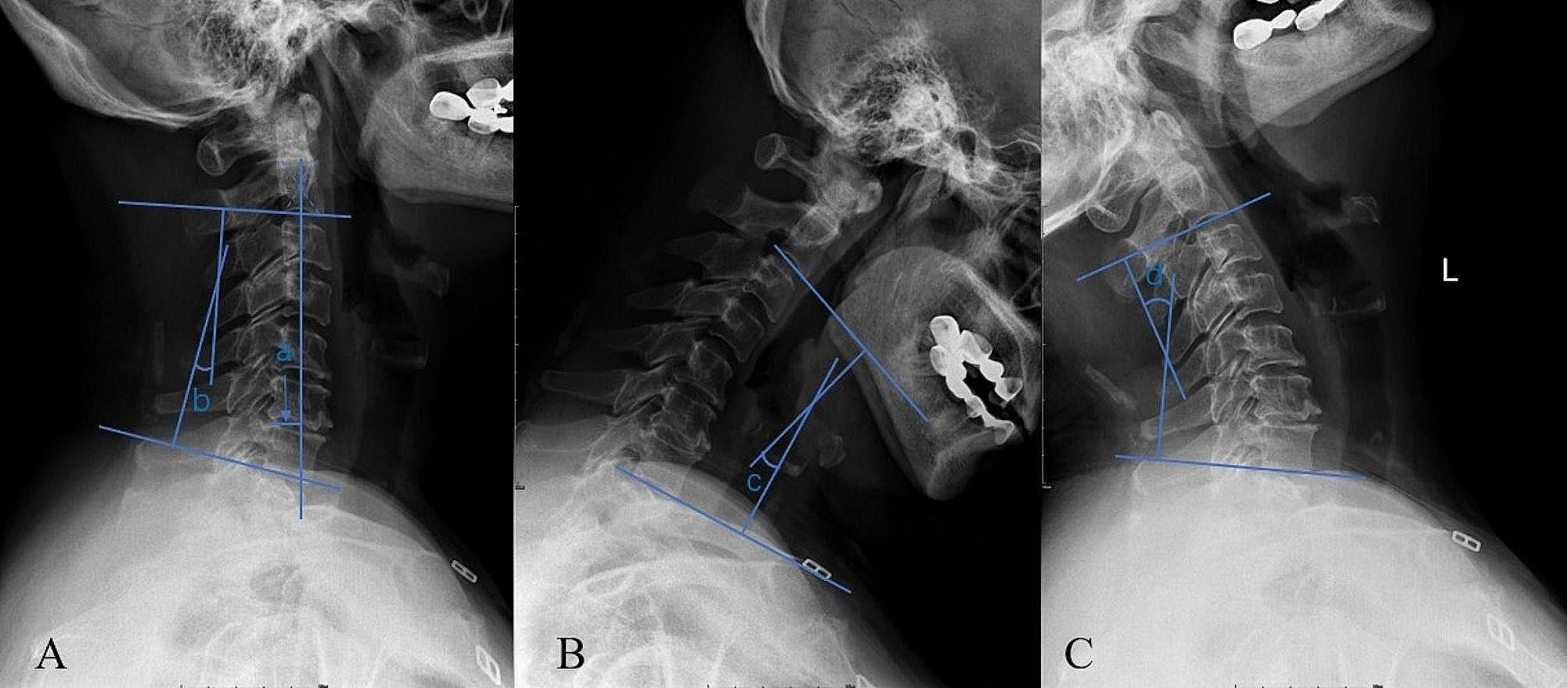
A, cervical X-ray in the neutral position: cSVA (a), the horizontal distance from plumbline dropped from the C2 vertebral body to the posterosuperior corner of the C7 vertebra; CL (b), the angle between the C2 lower endplate and the C7 lower endplate; B and C, cervical X-ray in the flexion and extension position: Flex CL (c) and Ext CL (d) refer to CL in flexion and extension positions. cSVA, cervical sagittal vertical axis; CL, cervical lordosis; Flex CL, cervical lordosis in flexion; Ext CL, cervical lordosis in extension
